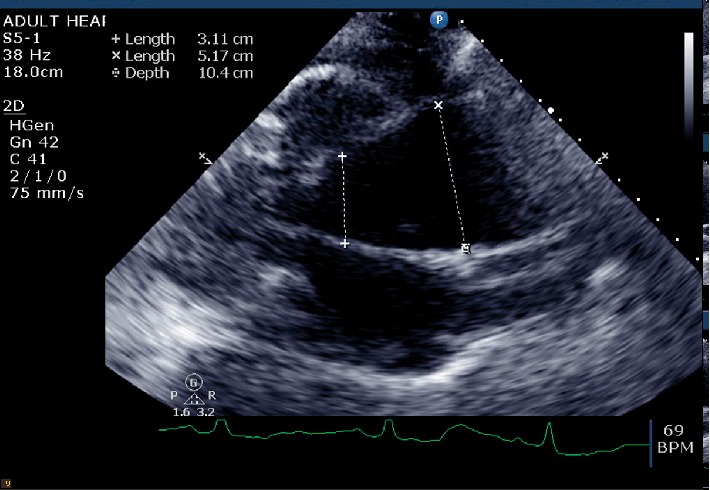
Parasternal long axis view showing the ascending aortic aneurysm.
